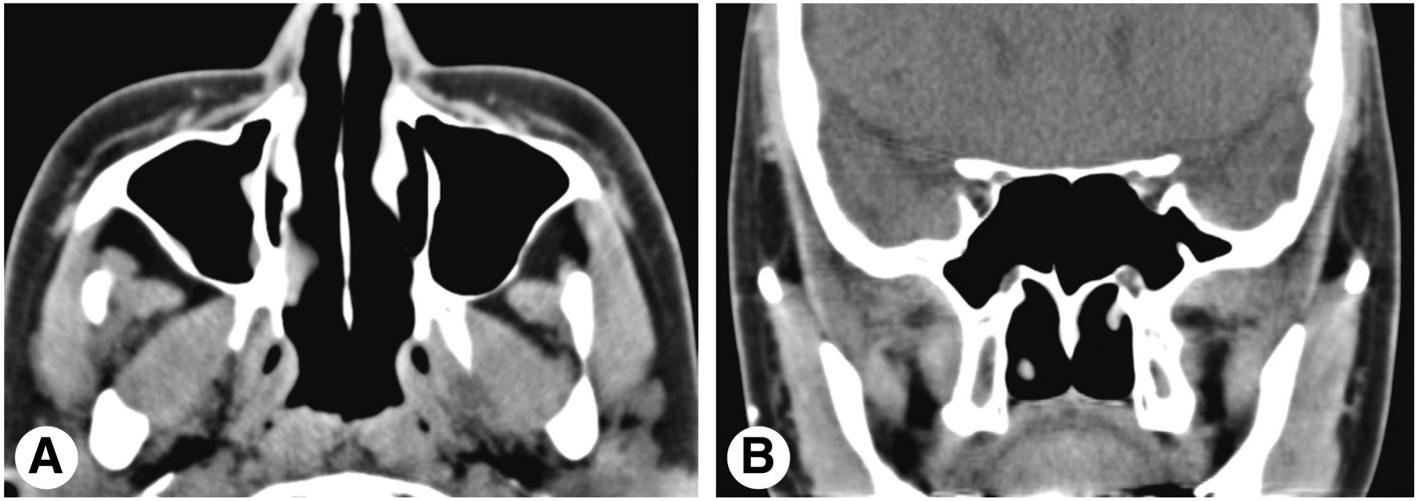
Postoperative computed tomography images of the paranasal sinus. Axial (A) and coronal (B) computed tomography images at 1 month postoperatively show complete resolution of the malformed middle turbinate and right maxillary sinusitis.
